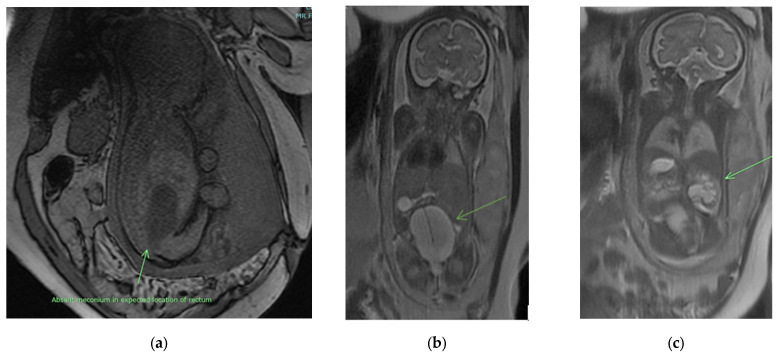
Prenatal MRI findings suggestive of a cloacal anomaly may include (as indicated by arrow): (a) absent meconium signal in the expected location of the rectum, (b) hydrocolpos with vaginal septum, and (c) hydronephrosis.
